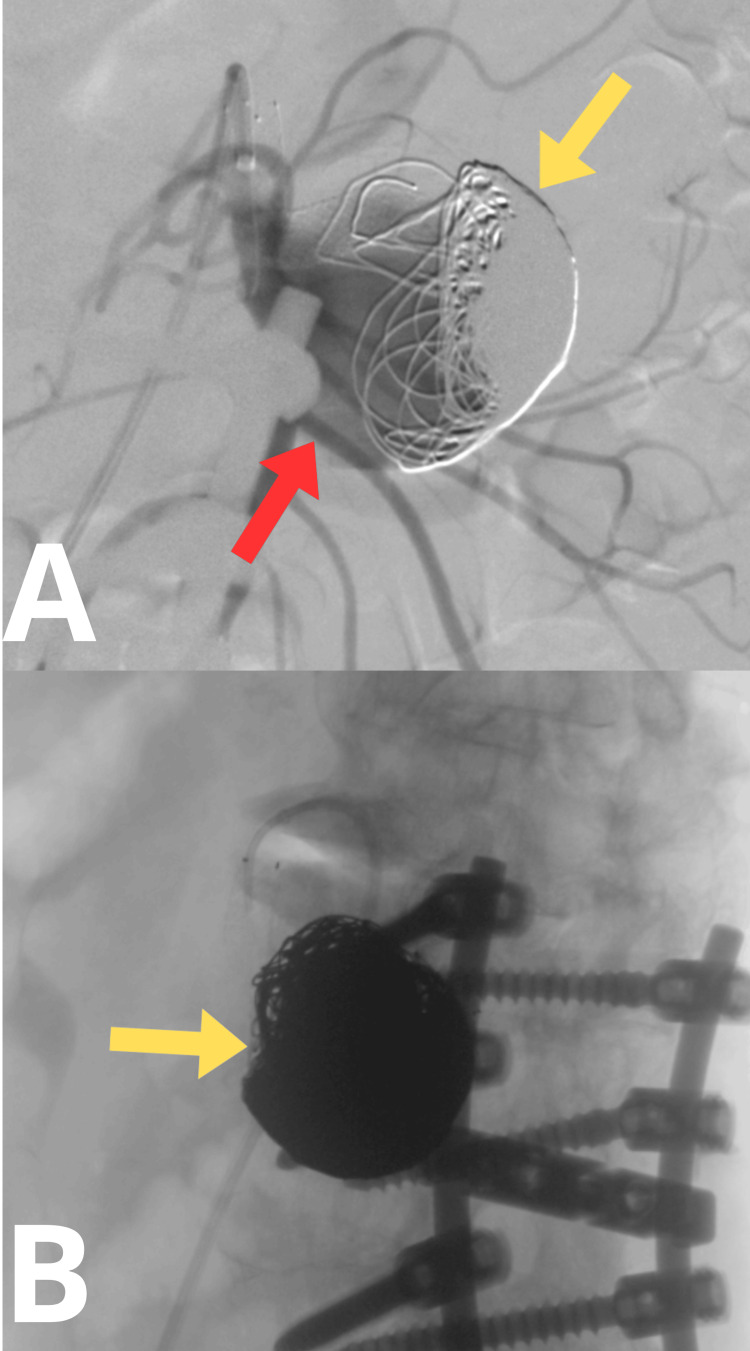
Intraoperative angio-CT. (A) Displaced embolization coils. (B) Complete aneurysm embolization. The yellow arrow indicates embolization coils. The red arrow indicates the filling portion of the aneurysmal sac.
